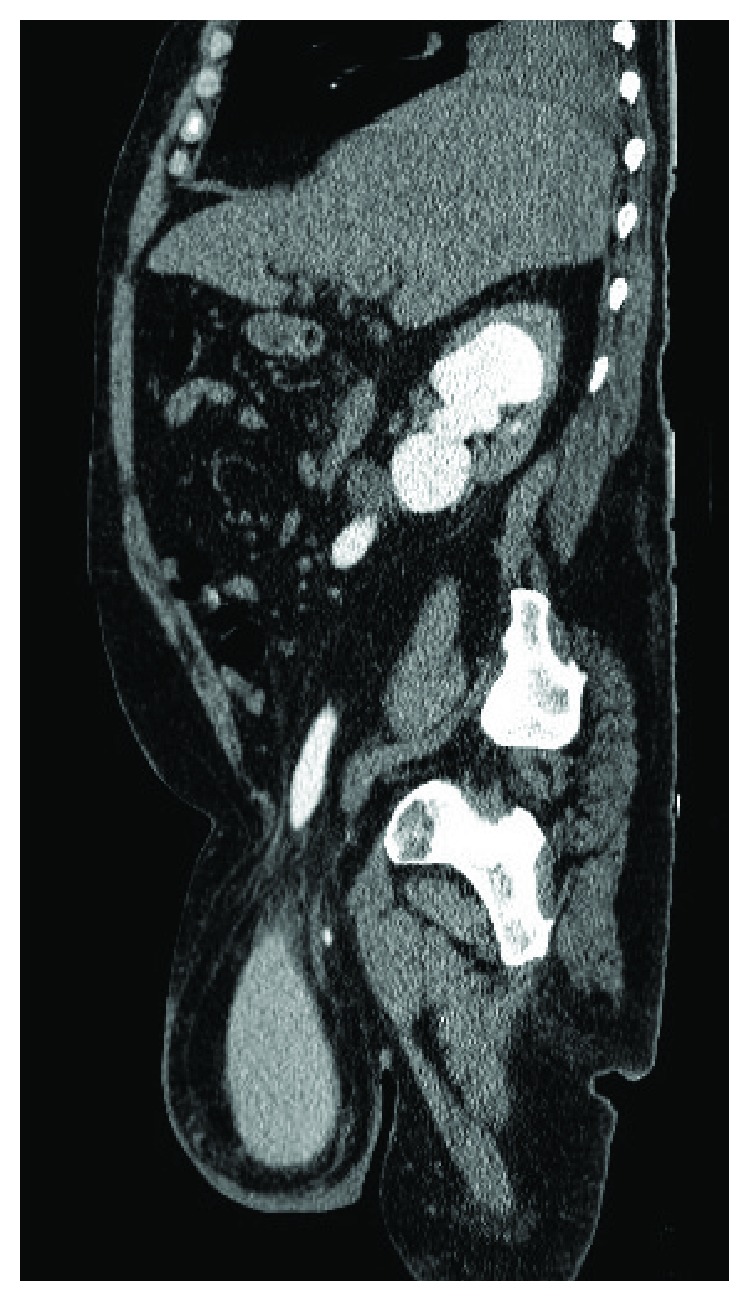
Abdominal-pelvic CT scan with endovenous contrast: right inguinal hernia, containing the whole urinary bladder and the right pelvic ureter dilated; sagittal reconstruction.
